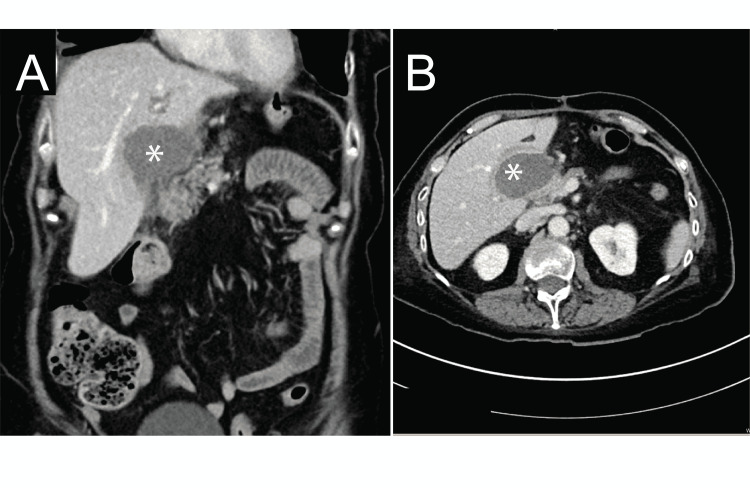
Abdominal computed tomographic (CT) scan. Abscess with complex fluid collection measured 5.3 x 3.6 x 5.8 cm^3^ (Asterix) in coronal (A), and axial view (B).
